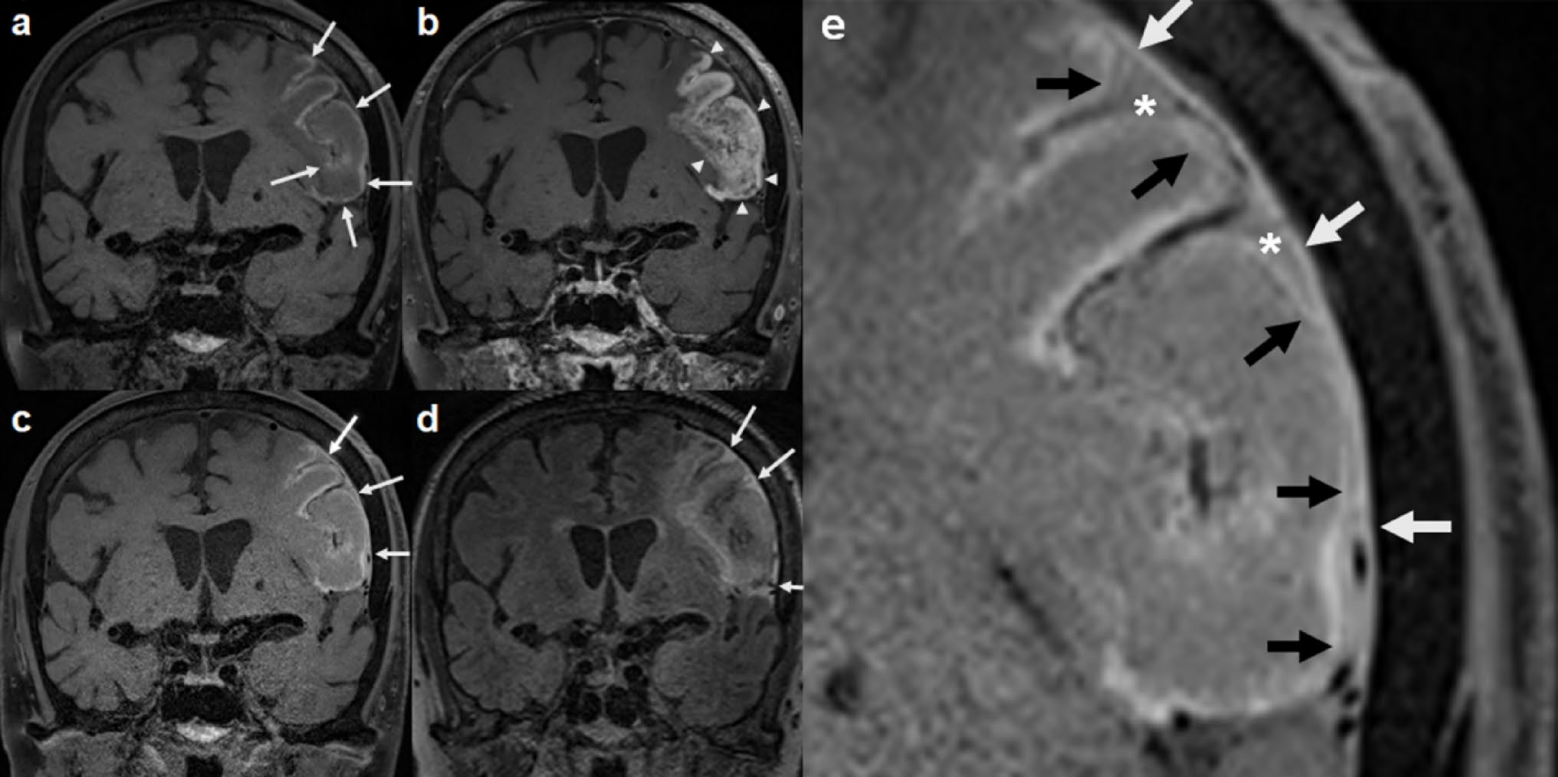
Representative images in a CVD patient (Case 2–CVD) with a large, subacute, left frontal lobe infarction. The NC DANTE T1-SPACE image a revealed regions of high signal intensity along the cortices, indicating hemorrhage or necrosis (arrows). The CE DANTE T1-SPACE image b acquired immediately after contrast injection showed contrast enhancement across the entire infarcted region (arrowheads). In the Delayed DANTE T1-SPACE image c obtained five hours after GBCA administration, the enhancement within the infarcted area had diminished, while new enhancement was observed along the dura mater adjacent to the infarct (arrows). Additionally, the subarachnoid space in that region exhibited contrast enhancement (c), which was obvious on FLAIR in the delayed phase (arrows) (d). Enlarged view of the Delayed DANTE T1-SPACE image e focusing on the infarcted region demonstrates enhancement along the dura mater (white arrows), pia mater (black arrows), and within the CSF space (asterisks)
